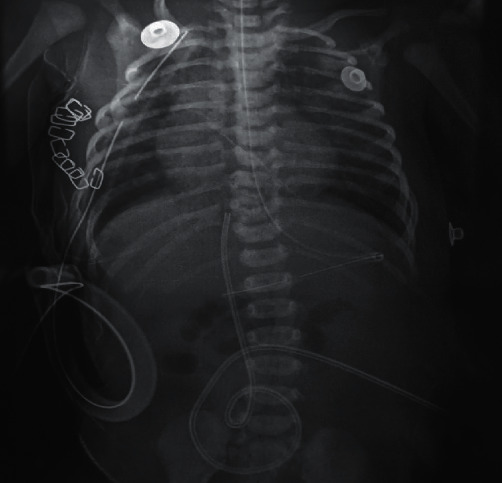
X-ray chest showed the nasogastric tube in the stomach after the successful repair of the TEF.
